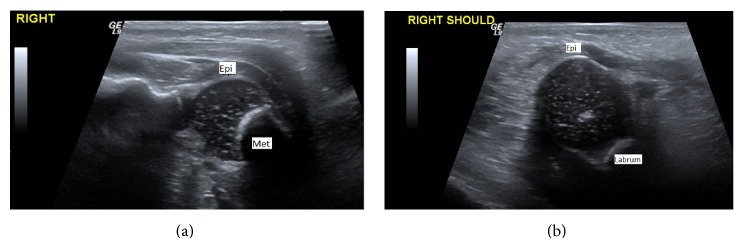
(a) Longitudinal sonogram of the right shoulder demonstrates the normal relationship of the epiphysis (epi) on the metaphysic (met). (b) Transverse sonogram of the right shoulder demonstrated the right humeral epiphysis within the right glenoid labrum.
